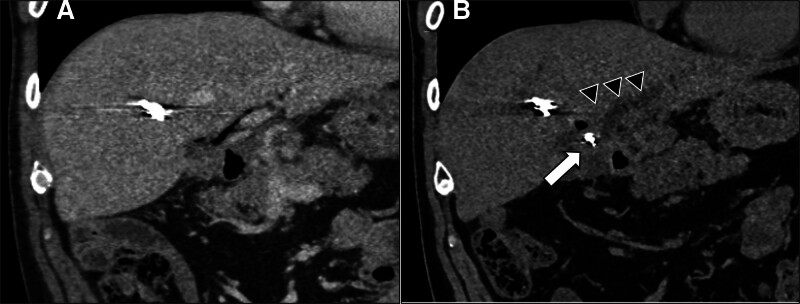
Comparison between the CT scan performed after coil embolization of the aneurysm 2 years ago and the CT scan upon admission. (A) CT scan performed after coil embolization of the hepatic artery (A8) aneurysm following biliary reconstruction surgery 2 years ago. (B) In the CT scan upon admission, partial migration of the coil to the hepatic hilum (➡) was observed, along with dilation of the intrahepatic bile ducts (▲). CT = computed tomography.
